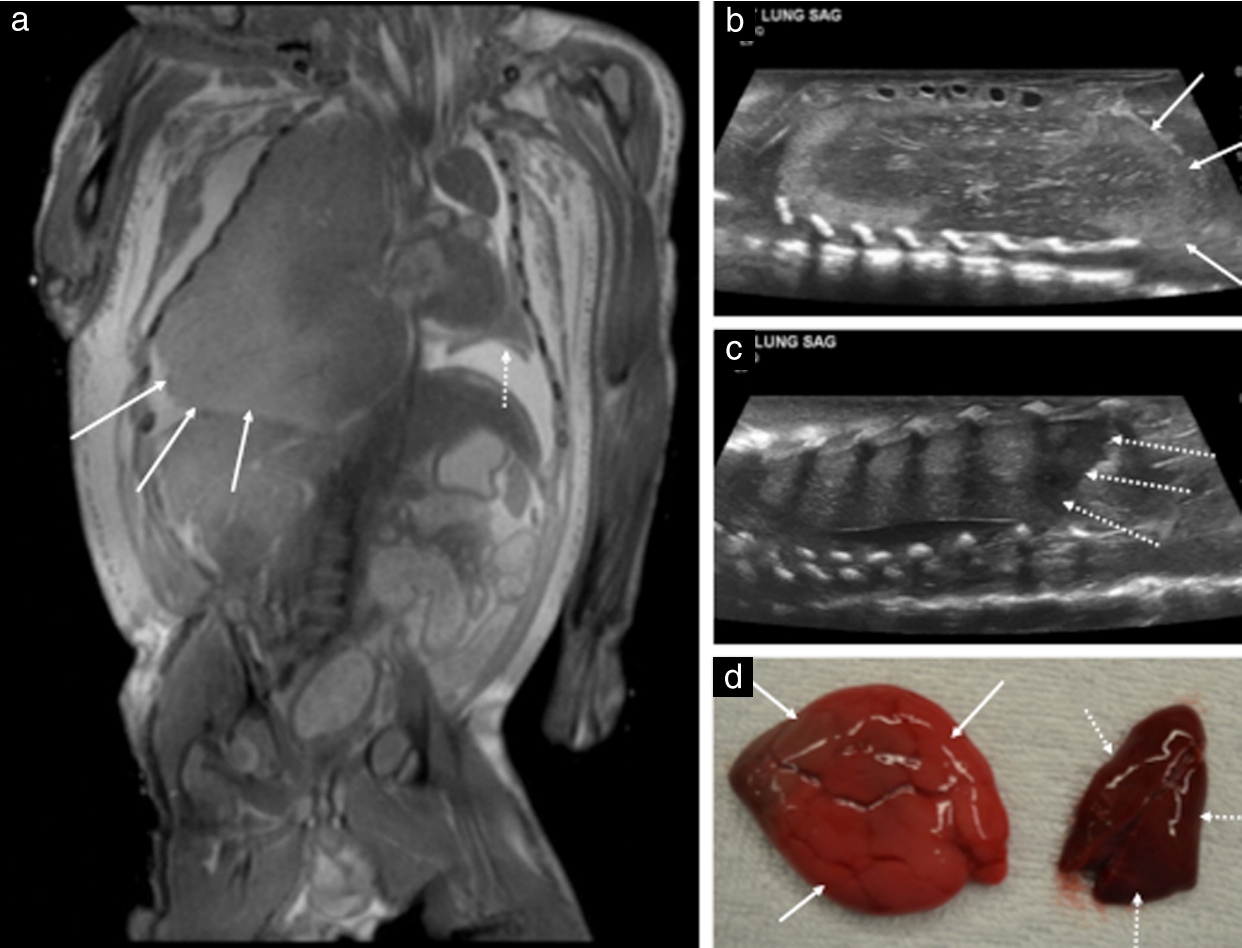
Postmortem imaging of right‐sided congenital lobar overinflation in 23‐week fetus: example of correct diagnosis on postmortem magnetic resonance imaging (PM‐MRI), but false negative (i.e. missed diagnosis) on postmortem ultrasound (PM‐US). (a) Coronal T2‐weighted PM‐MRI image of thorax and abdomen, demonstrating very enlarged right lung with bulging lower lobe (solid arrows) that displace inferiorly right hemidiaphragm. There is also mediastinal shift to left, with comparatively smaller left lung (dashed arrow). (b) Sagittal PM‐US image of right lung showing bulging inferior lobe (arrows) and heterogeneous internal lung parenchyma which was not reported or identified as abnormal at time of imaging. (c) Sagittal PM‐US image of left lung is provided for comparison, showing normal appearance of left hemidiaphragm (dashed arrows) with no overinflation of lung. (d) Macroscopic photographs of extracted lungs at autopsy, demonstrating differences in appearance between abnormal right lung (solid arrows) and normal left lung (dashed arrows).
